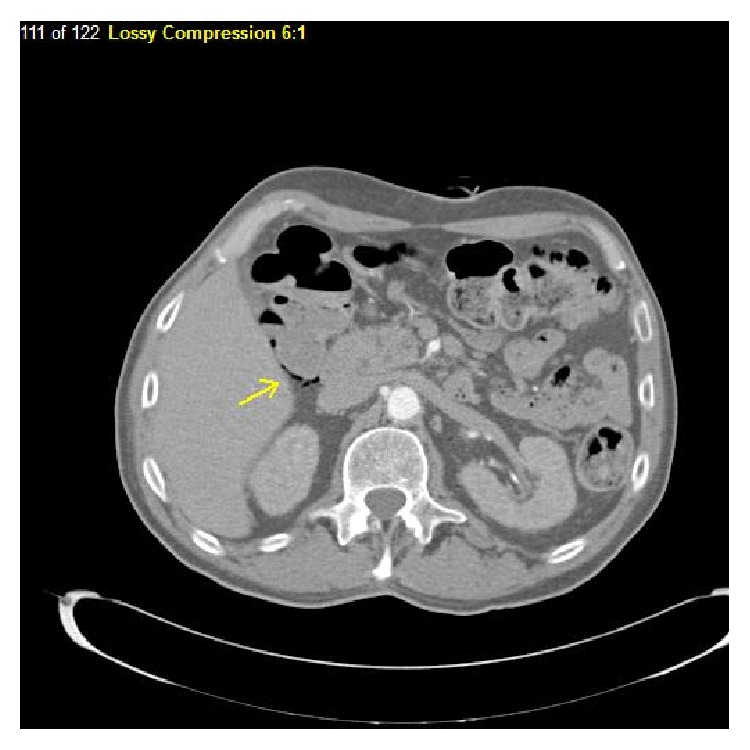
A CT image of dependent air in the bowel wall of Patient 1.
